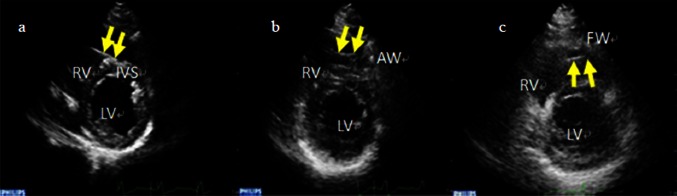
TTE determination of pacemaker lead position in parasternal short-axis view. The exact positions of the leads are documented (*yellow arrow*). a The lead is inserted into septum. b The lead passes over the septum and attaches into the anterior wall. c The position of the lead is anchored into the free wall
